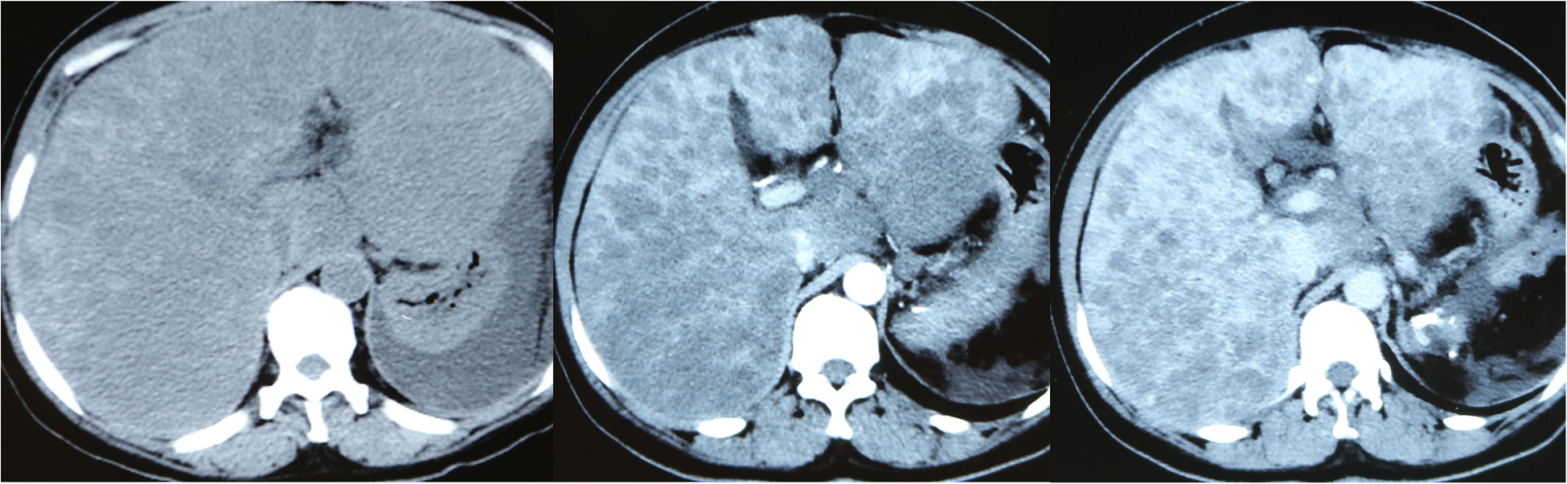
Abdominal computed tomography showed enlarged liver, irregular liver contours, heterogeneous hepatic parenchyma with diffusely distributed multiple hypodense focal lesions
